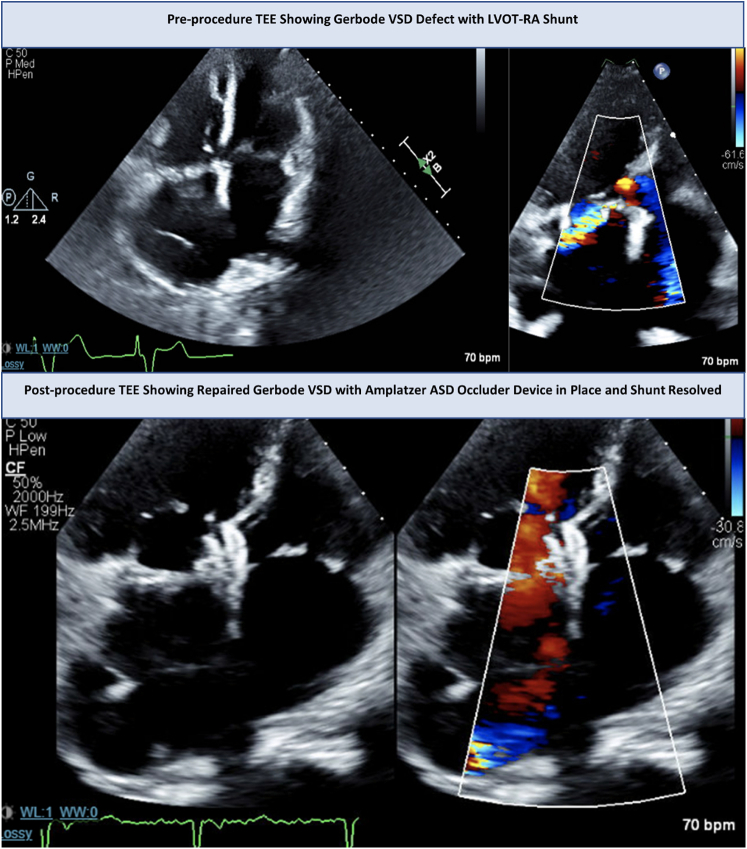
Preprocedure and Postprocedure Transesophageal Echocardiography ASD = atrial septal defect; LVOT-RA = left ventricular outflow tract–to–right atrium. TEE = transesophageal echocardiography
